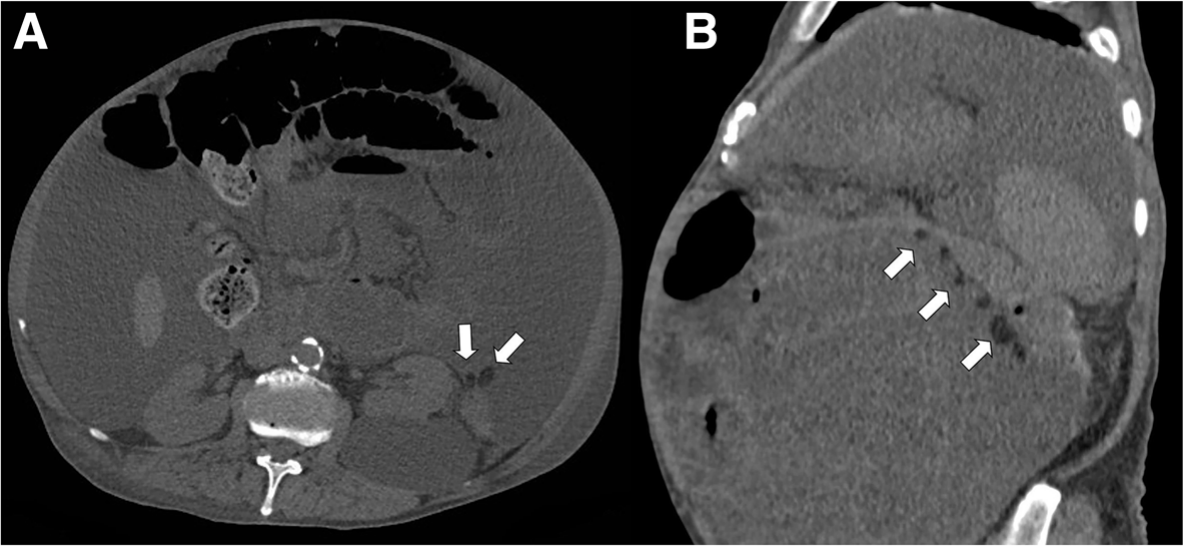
Axial (a) and sagittal reformatted (b) non-contrast CT images demonstrates massive ascites revealing the normal epiploic appendages (arrows), abutting the splenic flexure of the colon
